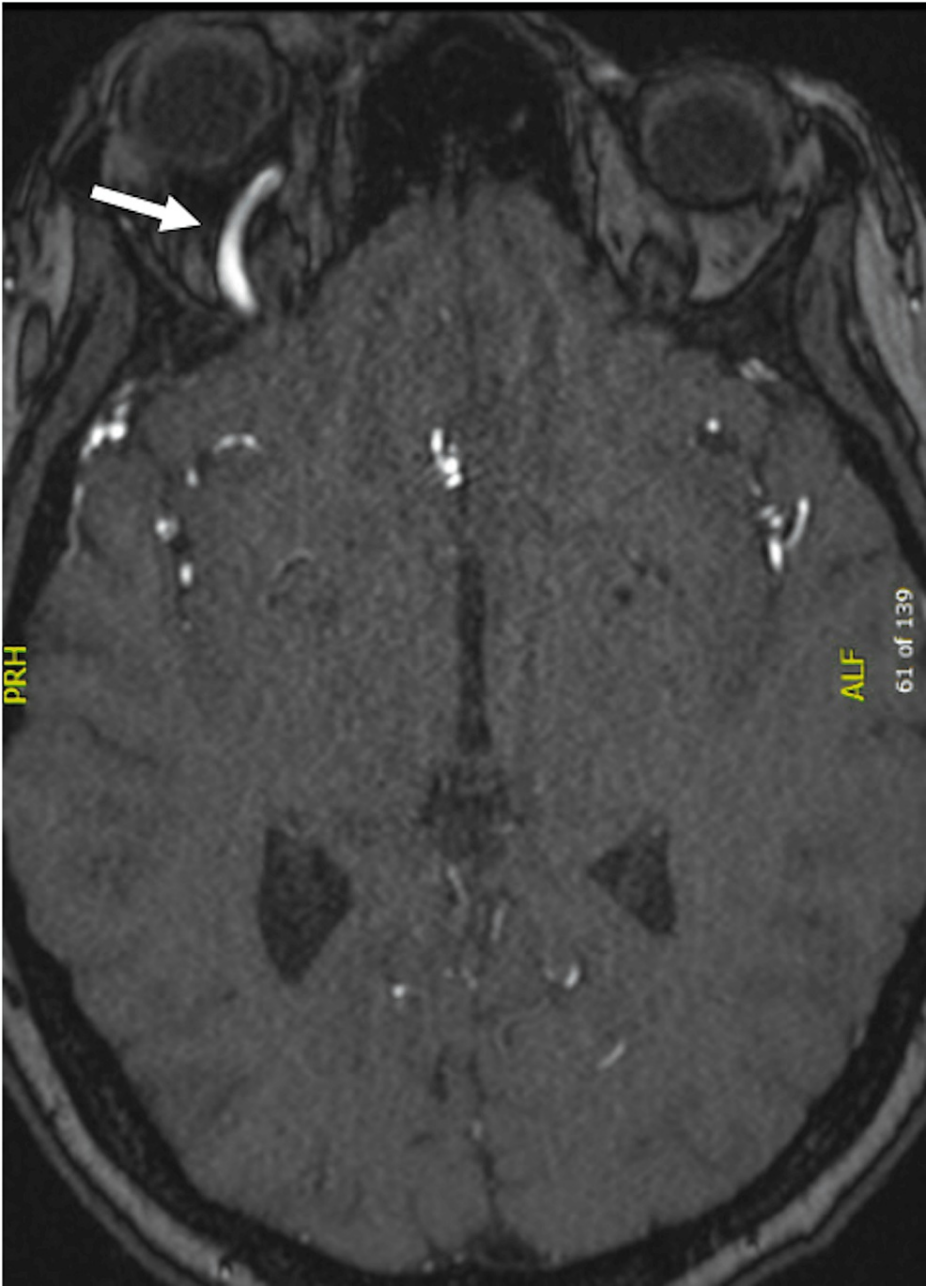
MRI brain angiography with contrast: TOF3D image showing increased flow-related signal in the dilated right superior ophthalmic vein (see arrow) as well as in the right cavernous and petrosal sinuses, suspicious for a carotid-cavernous fistula. MRI: magnetic resonance imaging
